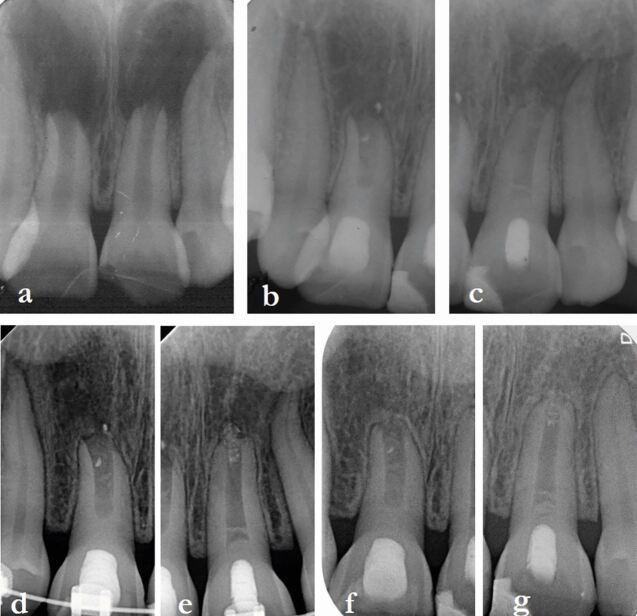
A 17-year-old female patient suffered crown fractures on her maxillary central incisors when she was about nine years old; she could not recall the exact trauma history. She had been asymptomatic since the trauma but had noticed that the teeth were discolouring. The teeth were both diagnosed with necrotic infected pulps and asymptomatic apical periodontitis. The treatment option selected was regenerative endodontic procedures for both teeth. a) Pre-operative periapical radiograph, July 2017. b, c) Tooth 11 and tooth 21, review radiographs February 2020. d, e) Review radiographs, October 2021, ten months after commencement of OTM. f, g) May 2023, review radiographs showing satisfactory biological outcomes for both teeth. Images courtesy of Dr Chee Wei Yeap
